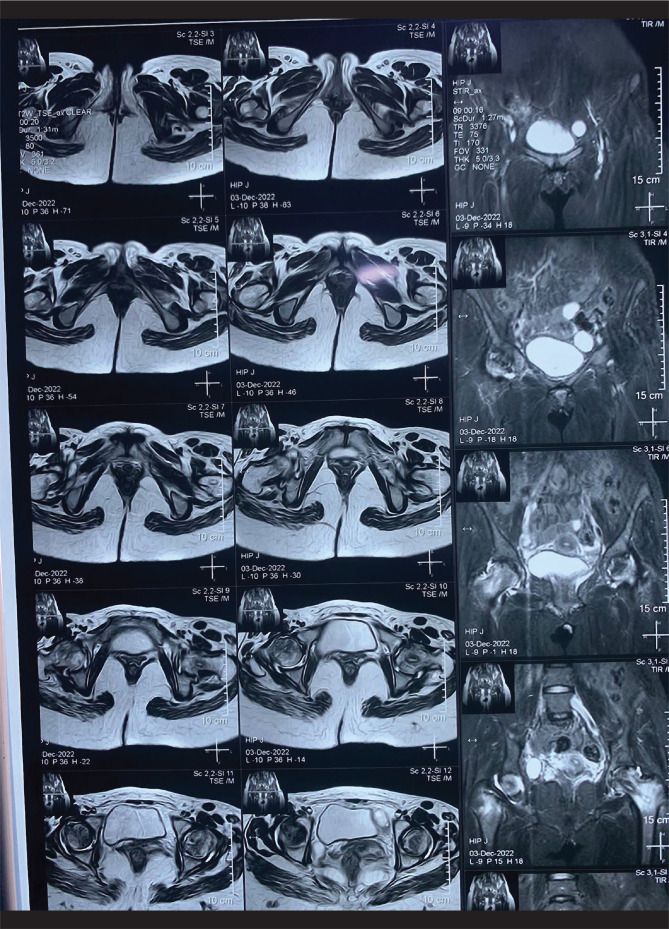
MRI changes in the follow-up period.
